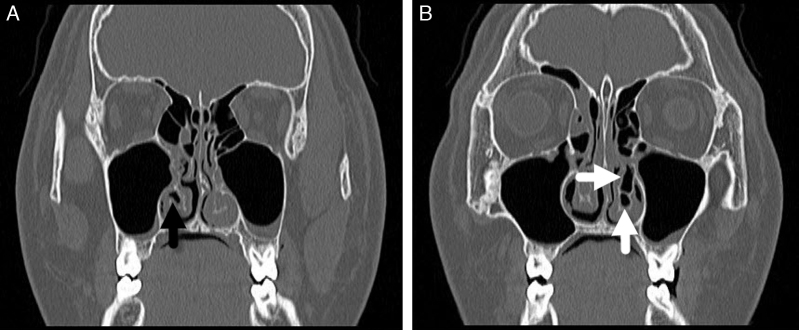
Computed tomography (CT) images of the patient. (A, B) Coronal view of CT images shows pneumatization of both the inferior turbinate and hypertrophy of the left inferior turbinate (black arrow, right inferior concha bullosa (ICB); white arrows, left ICB).
